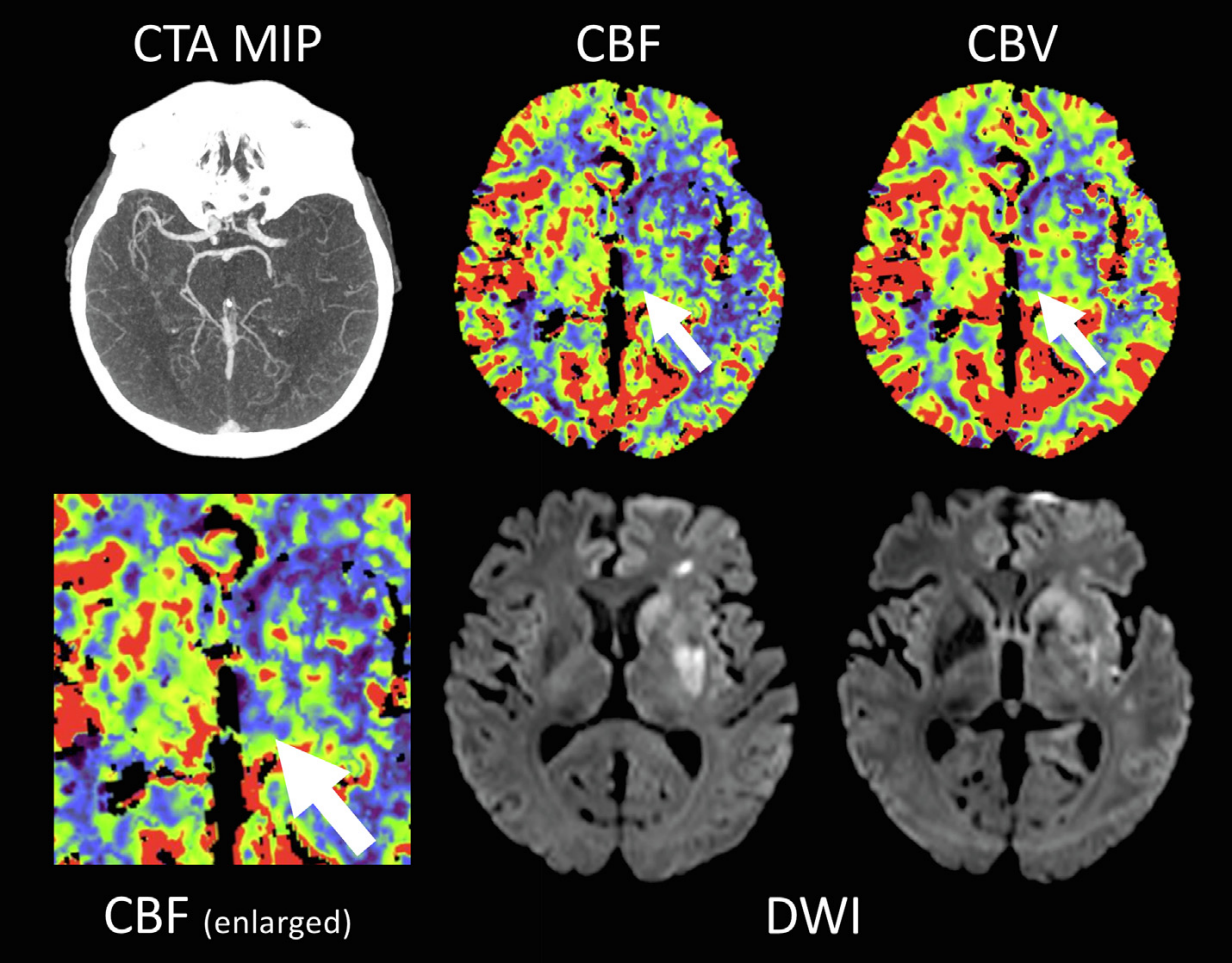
Example of a 74 year-old, male patient with left sided M1-occlusion (same as in Fig. 1). CTA presents proximal M1-occlusion on the left side with consecutive perfusion deficit in the left MCA territory on CTP. Additionally, marked thalamic hypoperfusion adjacent to the third ventricle is visible on the ipsilesional side (white arrows). Follow-up DWI after 6 days presents ischemic damage to the basal ganglia, but not to the thalamus. Also note absence of fetal PCA on CTA. Abbreviations: CTA, CT angiography; MIP, maximum intensity projection; CBF, cerebral blood flow; CBV, cerebral blood volume; DWI, diffusion weighted imaging; CTP, CT perfusion; PCA, posterior cerebral artery.
